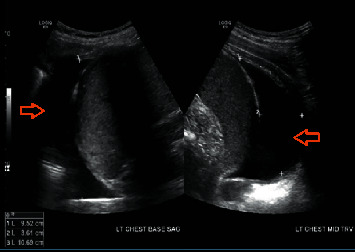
Ultrasound of the chest demonstrating a left simple pleural effusion measuring 150 mL.
